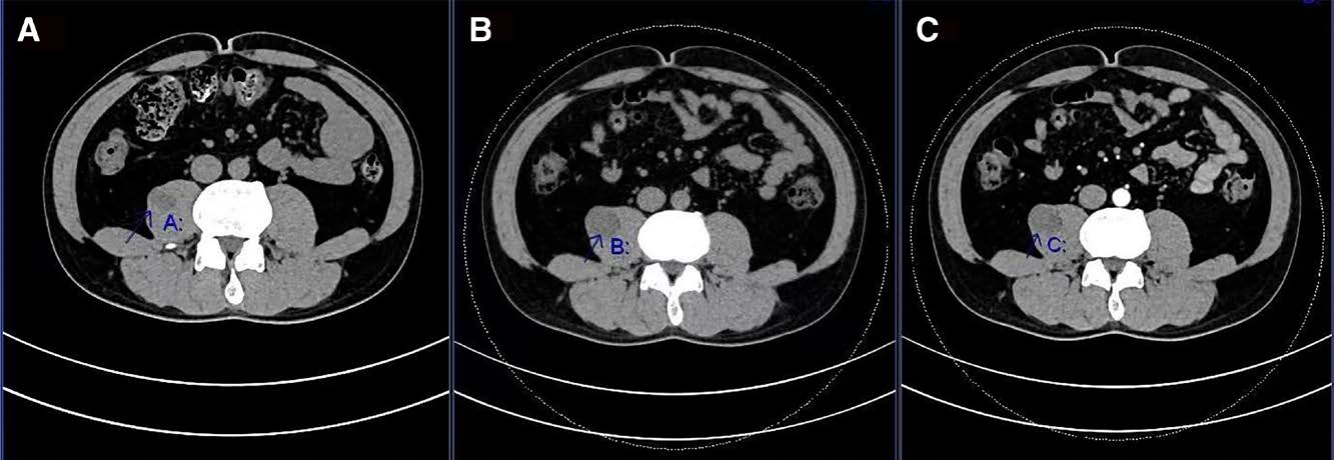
CT image. (A) in July 2021, the mass of psoas was well-defined and rounded; (B,C) in February 2024, the mass of psoas was enlarged and well-defined with no obvious blood supply. CT = computed tomography.
